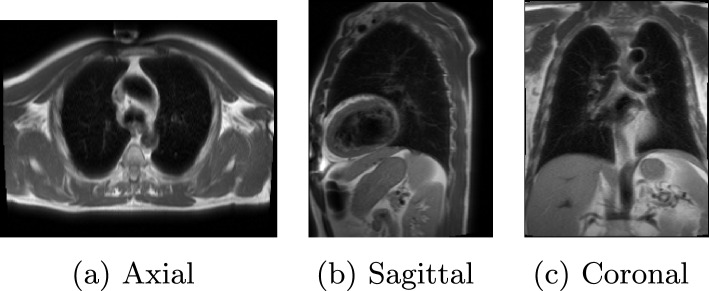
Original images from the sick dataset. a is Axial-view b is Sagittal-view while c shows a Coronal view of a chest MRI scan (one frame)
